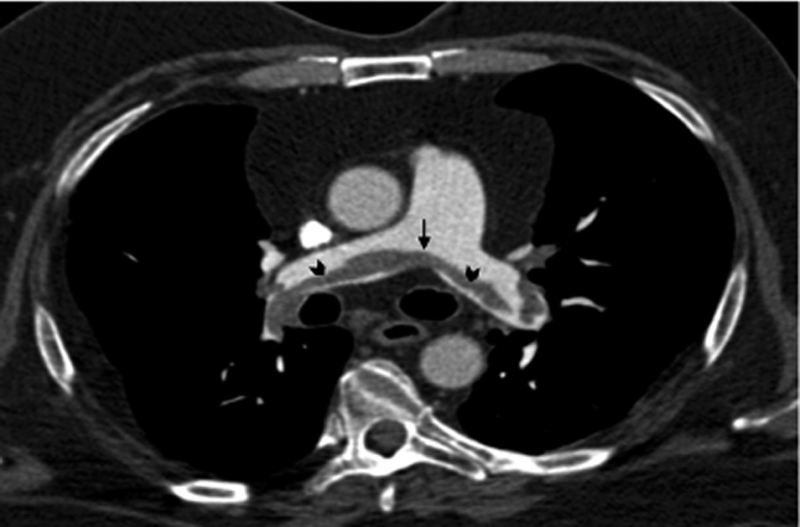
Chest CT angiography depicting a saddle PE. Image shows thrombus straddling the bifurcation of the pulmonary artery trunk (arrow) with extension into both main pulmonary arteries (arrowheads). CT, computed tomography; PE, pulmonary embolism.
